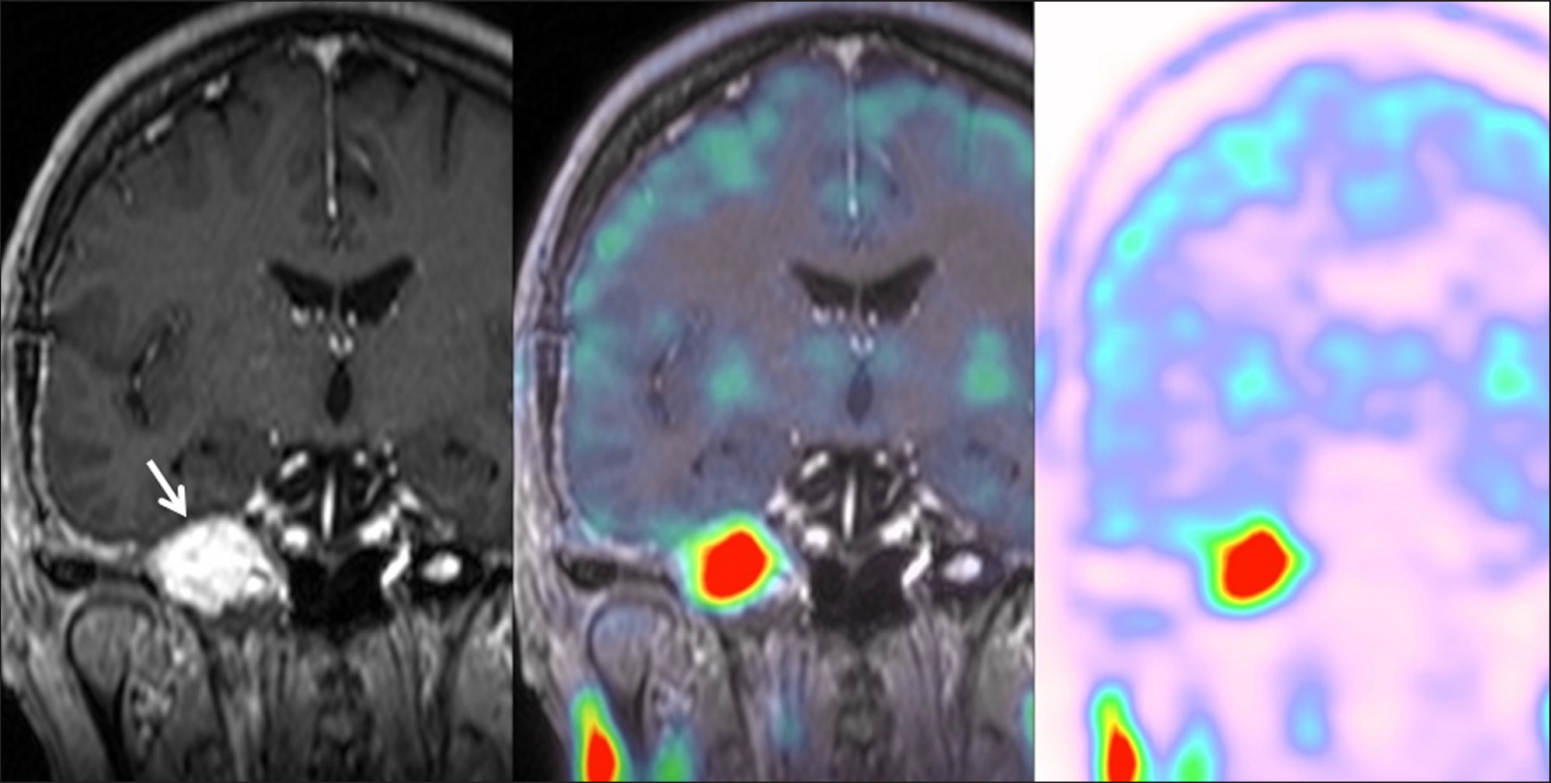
MET-PET/MRI shows the recurrence with a hypermetabolic activity (arrow).
